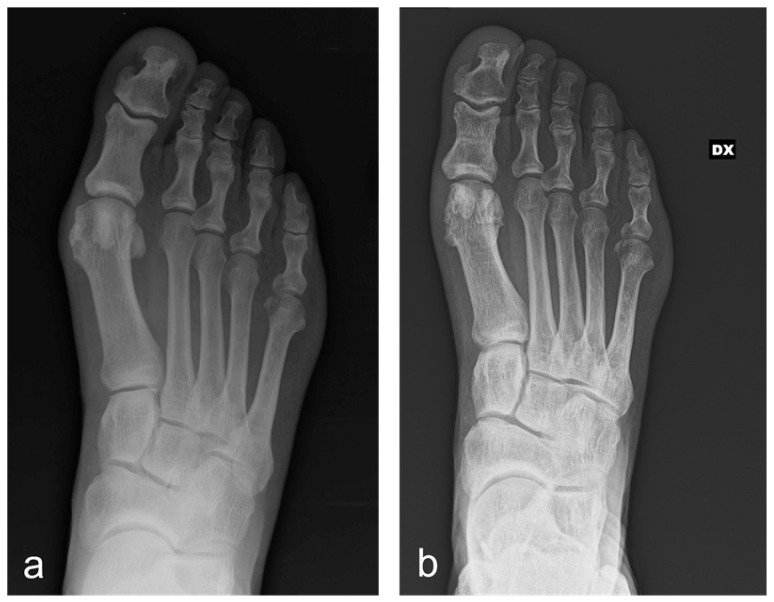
A 71-year-old man having undergone percutaneous Reverdin–Isham osteotomy, lateral release and Akin osteotomy for moderate HV correction: antero-posterior radiographic images at preoperative period (a) and 3-month follow-up (b), showing the complete healing of the osteotomies and the correction of the deformity. (DX: right foot).
